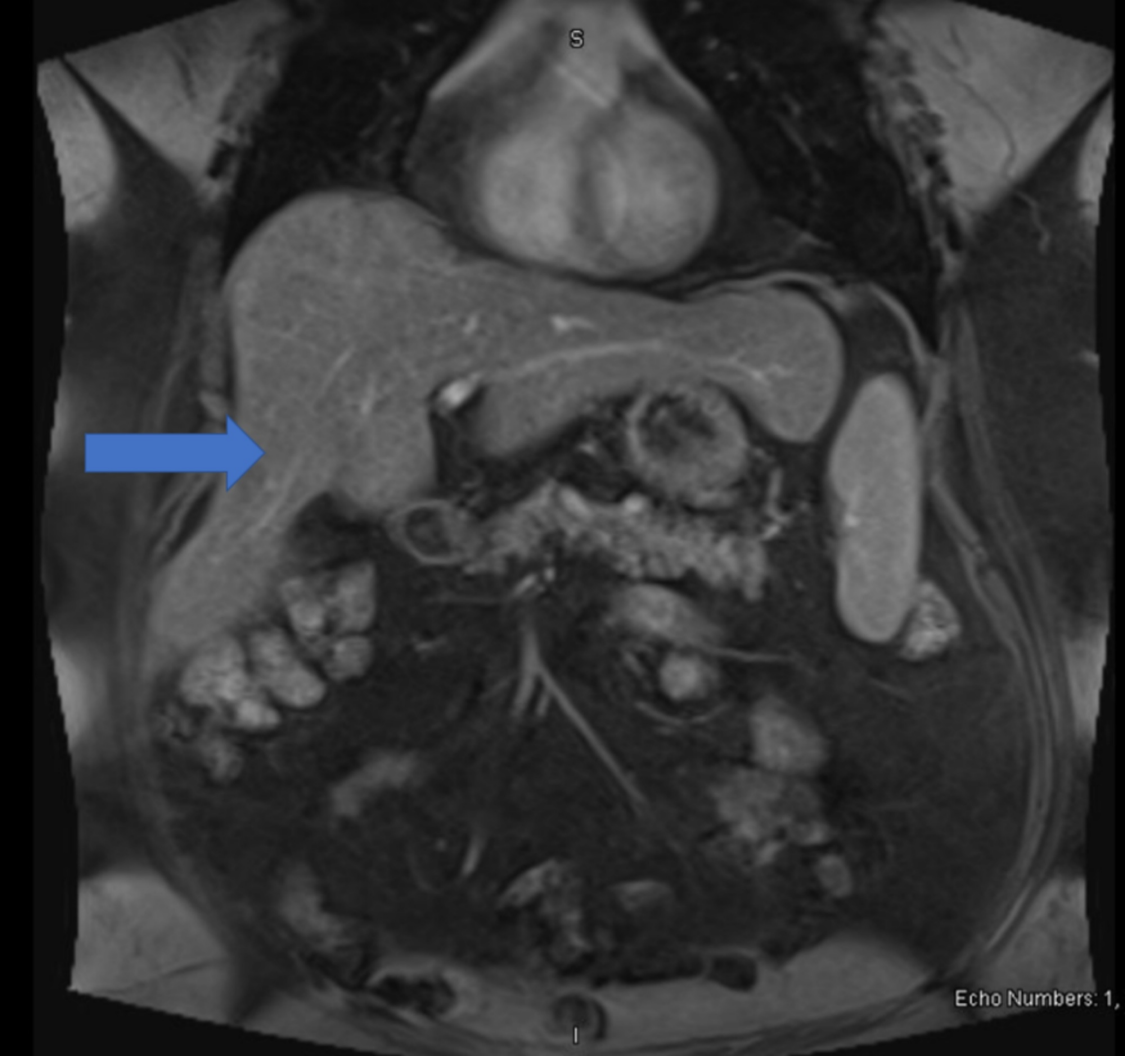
MRI abdomen - Hepatic enlargement with associated fatty infiltration change. - Postoperative changes status post cholecystectomy without intrahepatic or extra-hepatic biliary duct dilatation. MRI: magnetic resonance imaging
